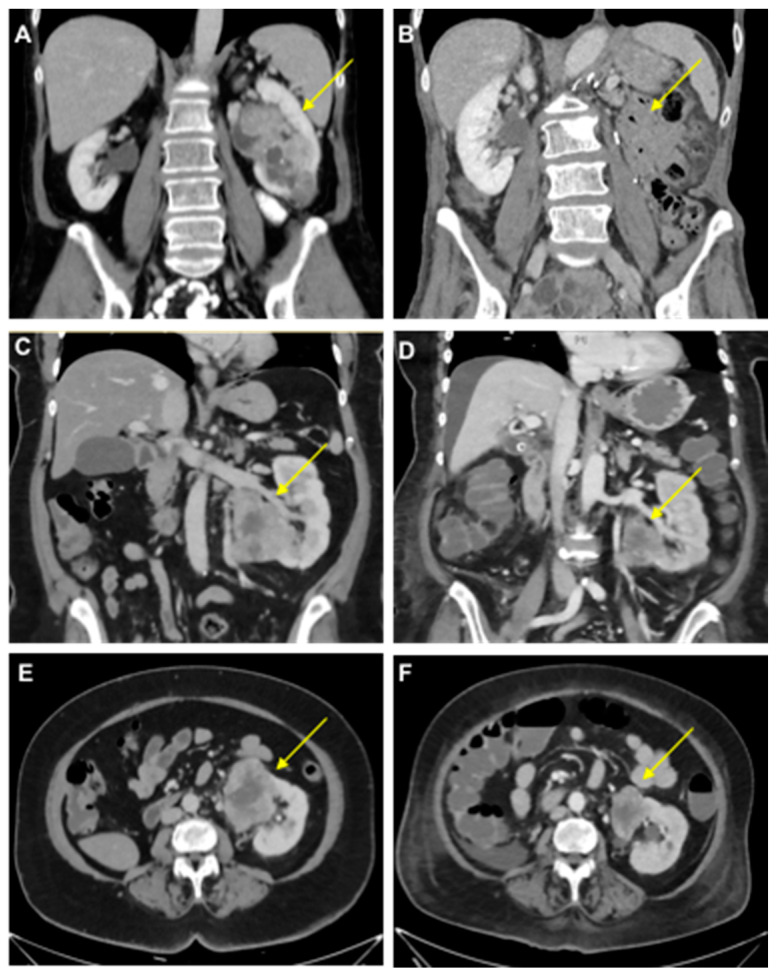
Clinical course of mRCC patients. (A,B) Computed tomography scans of the primary tumor in a 58-year-old female with oligometastatic mRCC before (A) and after (B) a left-sided tumor nephrectomy (Patient 1). (C–F) Tumor-bearing left single kidney in a 63-year-old female patient with congenital renal agenesia on the right side before (C,E) and after (D,F) the first cycle of therapy with ipilimumab/nivolumab with tumor regression (−31% after RECIST; Patient 2). Arrows indicate RCCs except in B.
